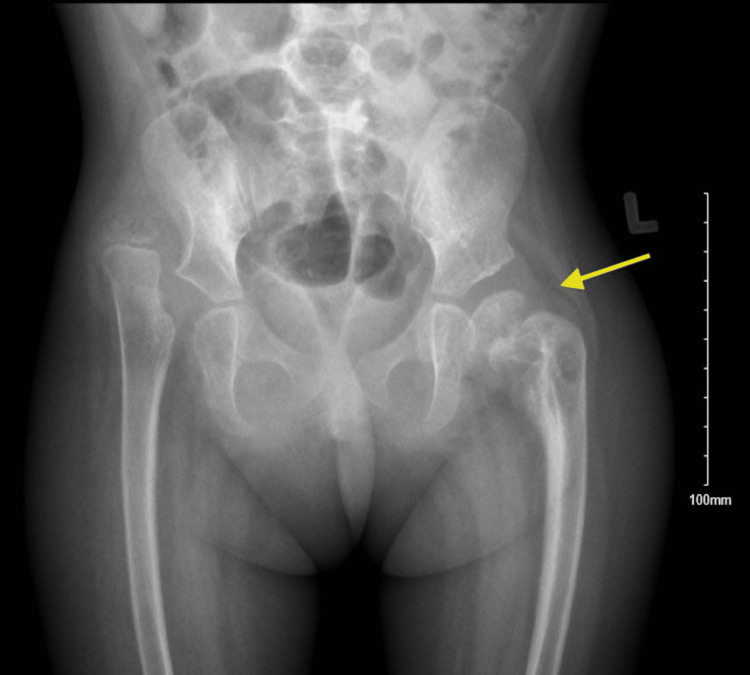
The X-ray of the patient taken 12 months post-injury
